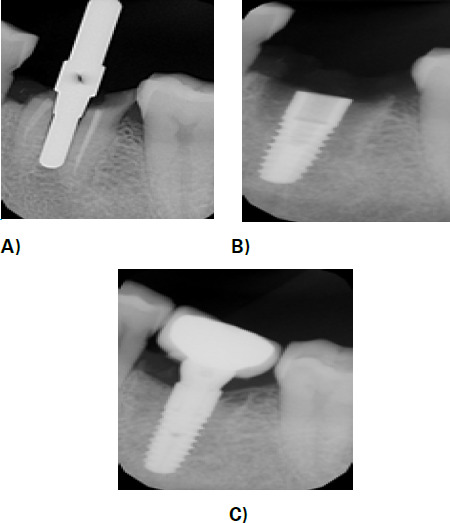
Radiographic image showing A) medial position achieved after initial osteotomy exactly between mesial and distal roots, B) implant placed after final drill in exact center point after roots removal, C) crown placed after 3 months.
